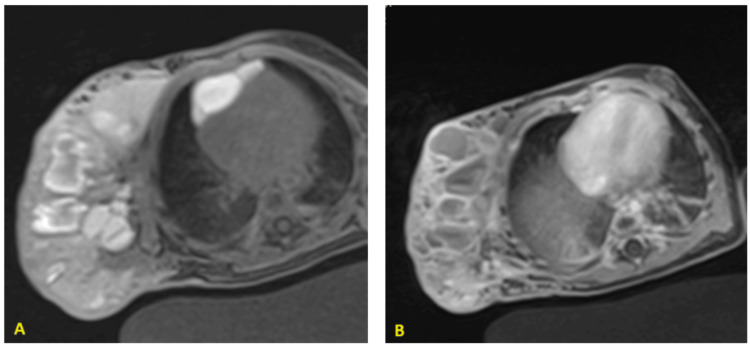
Axial MRI Sequences of the Upper Limb and Thorax A: Axial T1 sequence with fat saturation without contrast agent injection: Evidence of an anterior mediastinal lesion communicating with the previously described lesion and exhibiting similar signal characteristics B: Axial T1 sequence with fat saturation after contrast agent injection: Peripheral enhancement observed in some loculations, with progressive endoluminal enhancement noted in others
